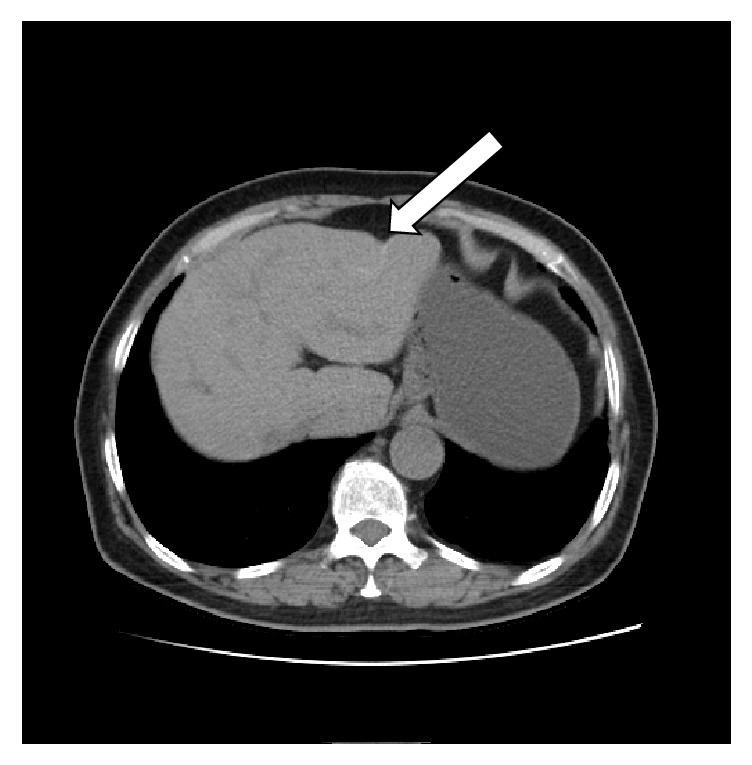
CT scan showing a linear calcification consistent with liver fibrosis due to* S. japonicum*.
